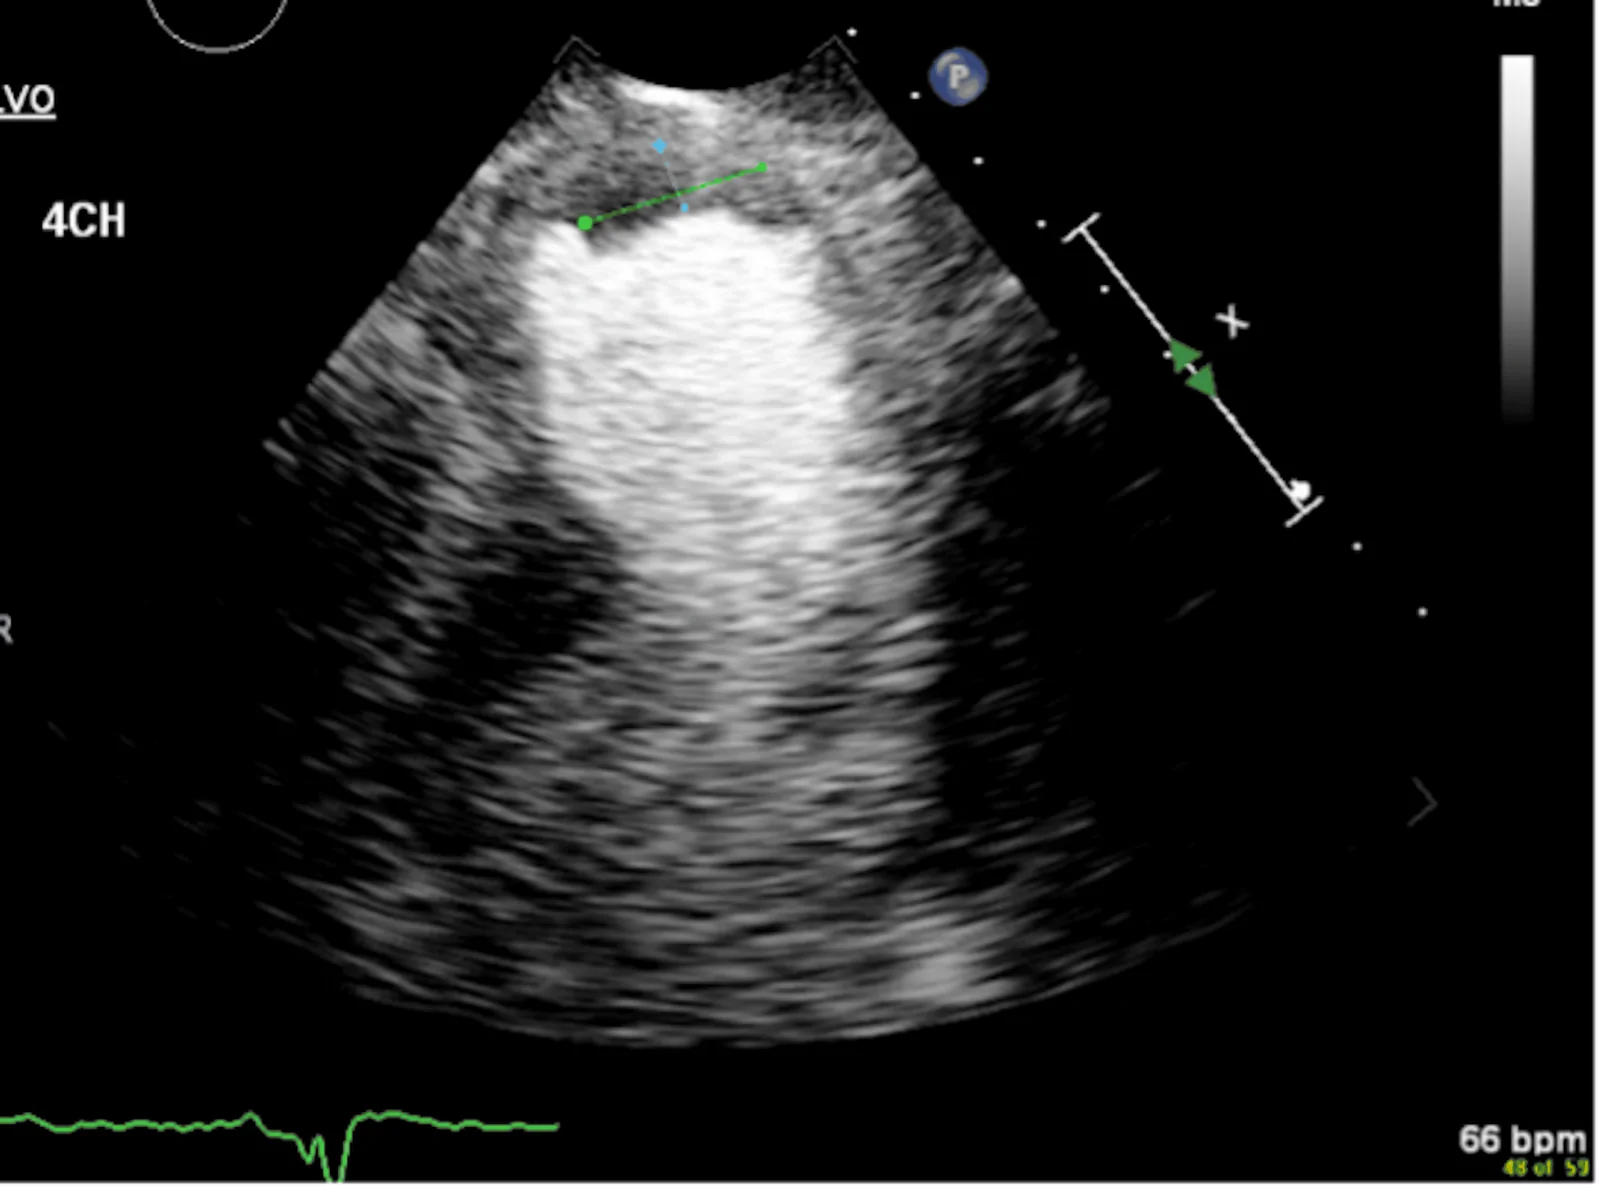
TTE in October 2025 with contrast (Definity) (A4C view) Left ventricle: mild to moderately decreased systolic function. Ejection fraction is mild to moderately decreased. The estimated ejection fraction is 40%-45%. Apex and apical segments are akinetic. Mild basal infero-septal severely hypokinetic. With contrast injection, an echolucency approximately 0.7 x 2 cm is visualized in the LV apex. TTE: transthoracic echocardiography, A4C: apical four-chamber, LV: left ventricle
